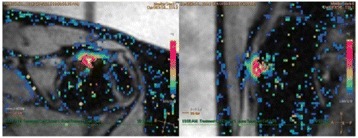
Representative MRI thermal imaging during HIFU treatment from a 30 W, 20s exposure (axial and sagittal views). Temperatures in the nidus reached >70 °C, indicating thermal ablation of the lesion
